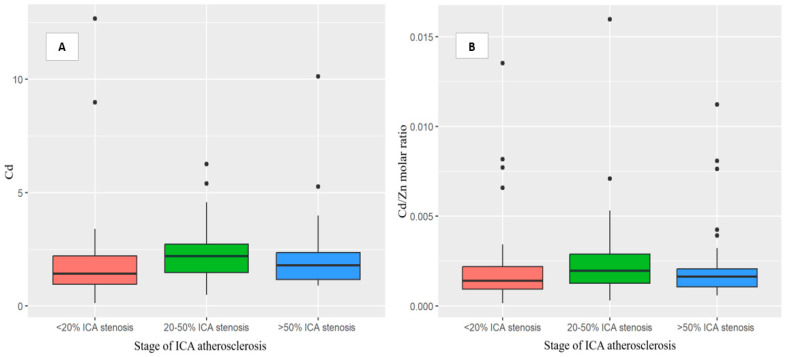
Box plot showing the findings regarding the Cd (A) and Cd/Zn (B) molar-ratio levels in acute-ischemic-stroke patients in relation to the stage of ICA atherosclerosis—B-mode ultrasound imaging using a standardized protocol or neck CT angiography. The patients with more advanced stages of ICA atherosclerosis had higher concentrations of the Cd and Cd/Zn molar-ratio levels, particularly those with 20–50% ICA stenosis. Abbreviations: Lead, Pb. Cadmium, Cd. Copper, Cu. Selenium, Se. Zinc, Zn. Internal carotid artery, ICA. Computed tomography, CT. Acute ischemic stroke, AIS. *p*-values < 0.05 showed statistical validity.
